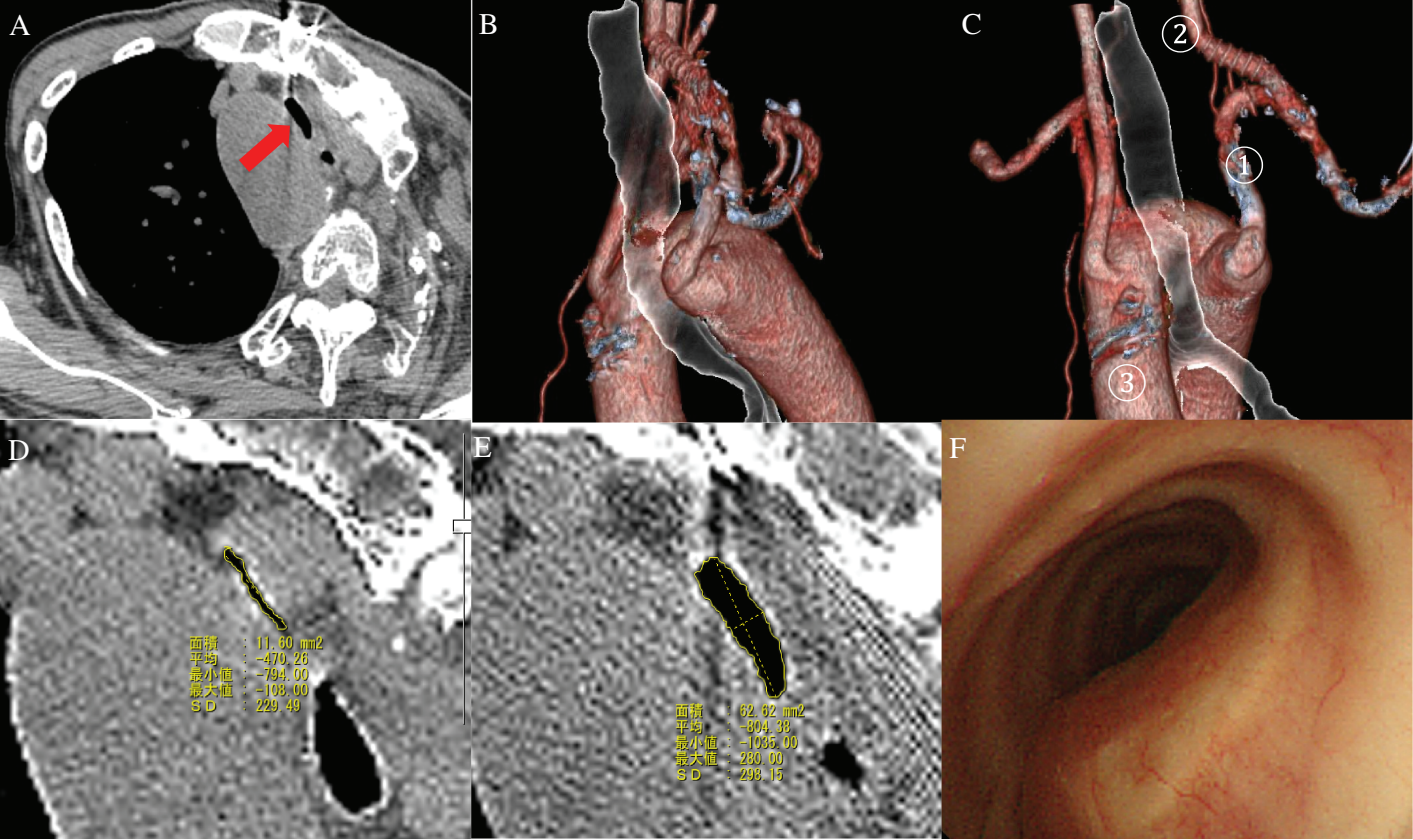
(A) Chest CT (mediastinal window) at 29 days after the bypass operation revealed expansion of the sectional area of the tracheal stenosis (arrow). (B, C) Chest CT with 3‐dimensional image reconstruction at 29 days after the bypass operation showed tracheal lumen dilation. (①: the left common carotid artery, ②: the left subclavian artery, ③: the right aortic arch.) (D) Chest CT (mediastinal window) revealed a pre‐operative sectional area of the tracheal stenosis of 11.60 mm^2^. The airway cross‐sectional area was measured using CT image customizable software (DICOM viewer‐EV insite R; PSP corporation, Tokyo, Japan). The most constricting area was manually indicated on the image. If the image of the most constricting area was indicated obliquely, the image was reconstructed to horizontally show the most obstructing area. The area was then calculated by tracing the most obstructing area in EV insite R. The percentage of the airway constriction was calculated by comparing the minimum cross‐sectional area with the cross‐ sectional area of nearby trachea (3 cm upper the constricted portion). A LungPoint® view is shown. The VBN system automatically indicated the distance to the peripheral end and proximal end of the target lesion. It also indicated the distance between virtual tip of the bronchoscopy and bronchial wall of the target in the view. From the view of this figure, the proximal end of the target is 7 mm, the peripheral end of the target is 15 mm, and the distance to the target bronchial mucosa is 4.3 mm. The puncture point is marked by white point in this view (i.e. center of the traced target lesion). (E) Chest CT (mediastinal window) revealed a post‐operative sectional area of the tracheal stenosis of 62.62 mm^2^. (F) Bronchoscopic findings at 34 days after bypass surgery showed improved healing of bronchial ulceration and maintenance of tracheal lumen dilation.
